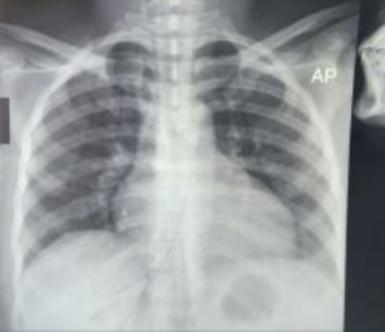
Normal erect chest X-ray excluding free subdiaphragmatic air.
